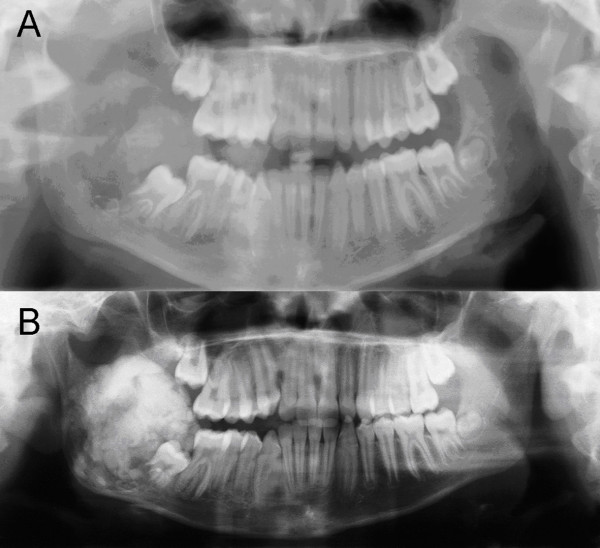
A: Panoramic radiography at first presentation: A large radiolucent lesion with irregular borders infiltrating the right ramus of the mandible. Displacement of tooth 47. B: Panoramic radiography after 3 months: The growth of the tumour mass is visible with a striking increase of radio-opaque structures.
